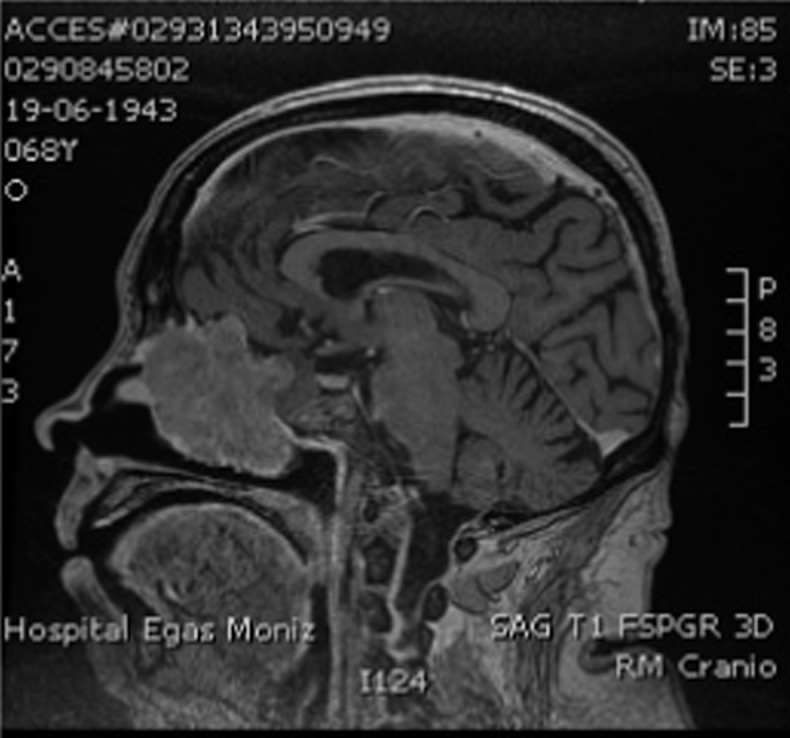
Cranial MRI showing a large mass on the right nasal sinuses.
